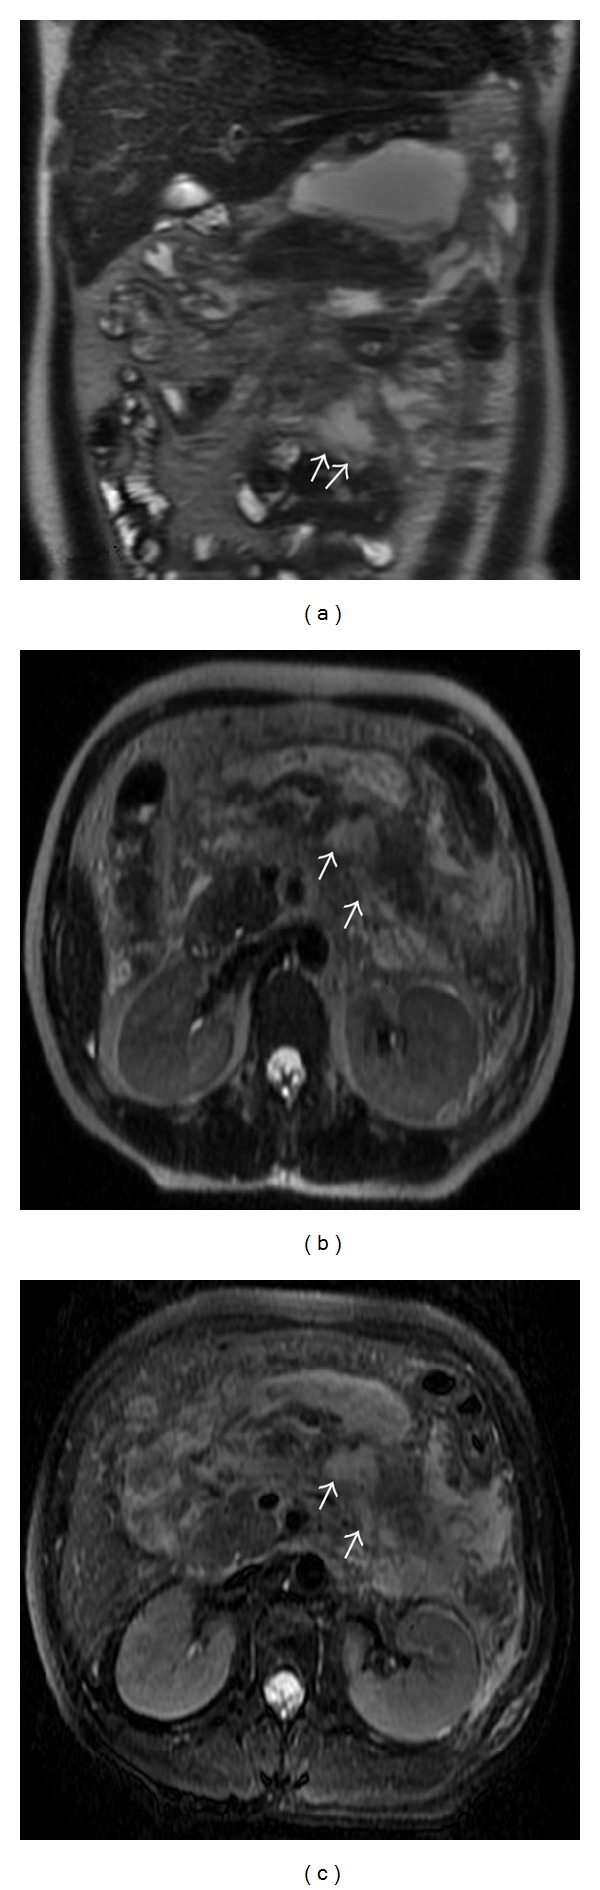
A 43-year-old female with severe AP. The mesostenium showed edema, thickening, and effusions on a coronal SSFSE T2-weighted image (a), on axial SSFSE T2-weighted image (b), and on axial SSFSE T2-weighted with fat suppression image (c).
